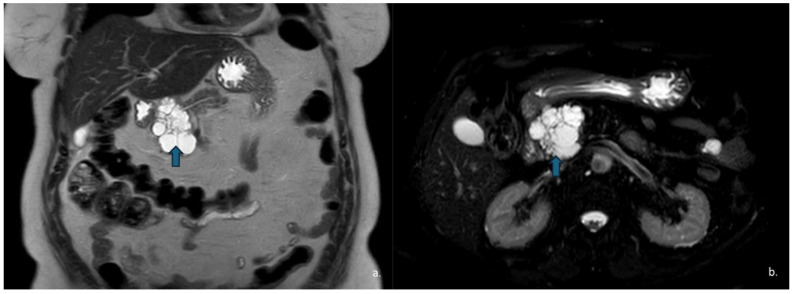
MRI of a 51-year-old woman. Coronal T2-w HASTE (a) and axial T2-w SPAIR (b) sequences show a hyperintense lesion (arrows) in the pancreatic head with a “cluster” morphology. Endoscopic ultrasound confirmed the diagnosis of a macrocystic serous cystadenoma.
